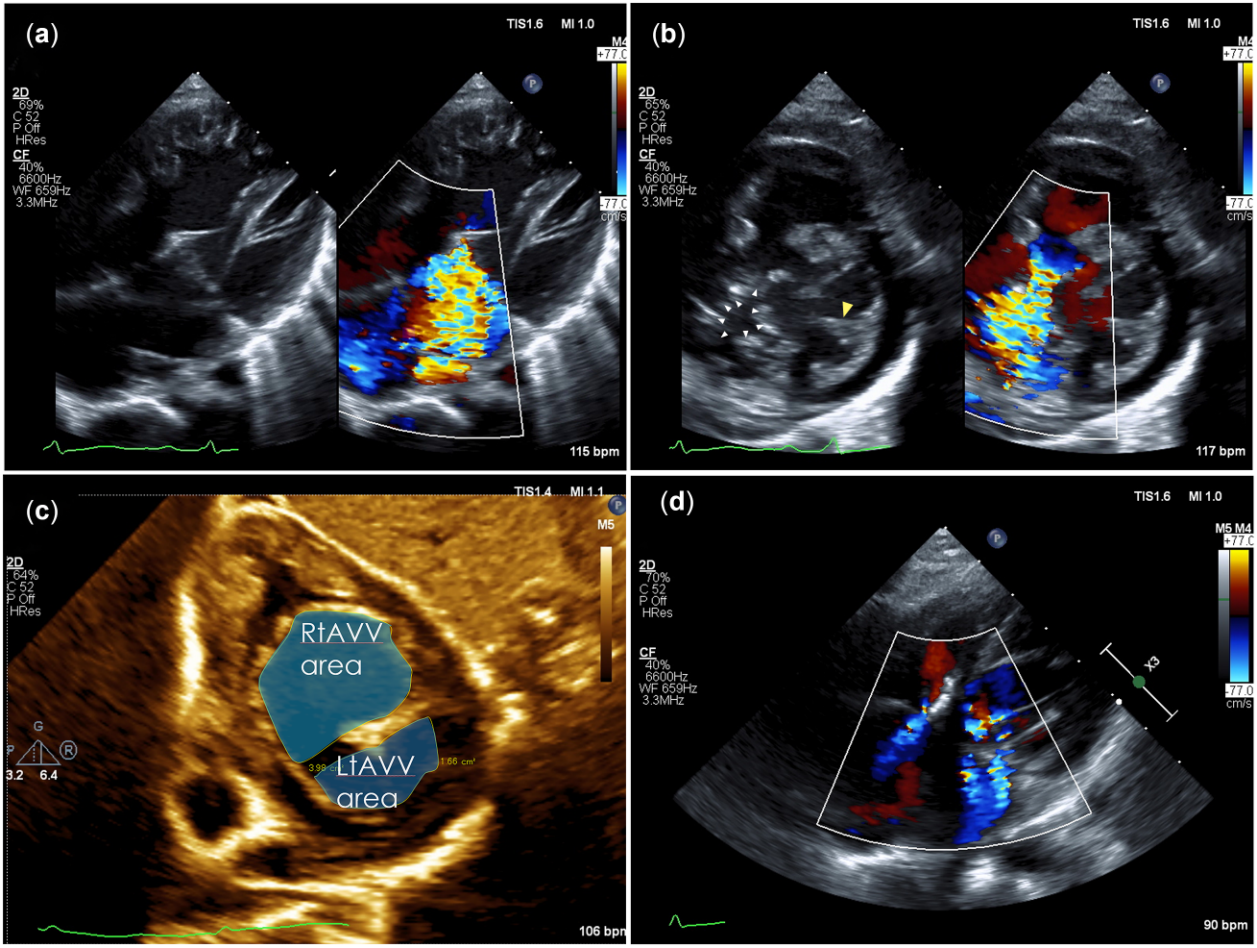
Preoperative echocardiography revealed severe right atrioventricular valve (AVV) regurgitation (annulus size 123% of normal) (a). Extensive gap between leaflets (b, white arrowheads). Marked hypoplasia of the left mural leaflet and single papillary muscle (yellow arrowhead) in the left atrioventricular valve (annulus size, 96% and 59% of normal on parasternal long-axis, four-chamber view, respectively). The modified AVV index was 0.29 (c). Echocardiography at 14 months postoperatively showed moderate left- and right-sided atrioventricular valve regurgitation (d).
